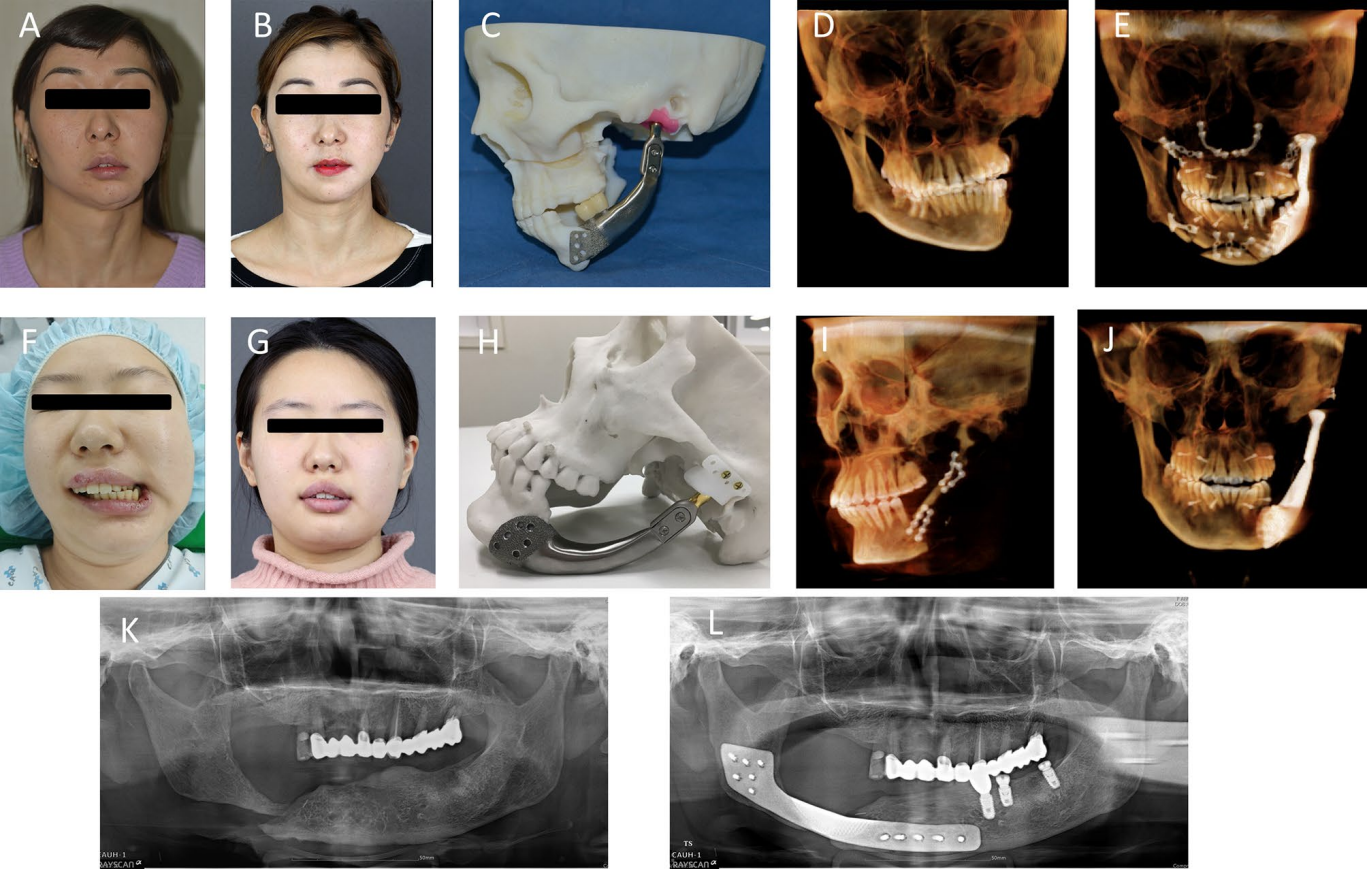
Titanium reconstruction of tumor-induced mandibular defects. (A) A 25-year-old female patient was referred for the reconstruction of the left mandible and correction of facial asymmetry; (B) Postoperative photograph of the female patient. (C) A titanium three-dimensional (3D)-printed mandible was fabricated. The two abutments that faced the central fossa of the maxillary molar were designed for prosthetic restoration. Temporary crowns were also 3D-printed. (D, E) Preoperative and postoperative 3D reconstructed cone-beam computed tomography (CBCT) images. Reproduced from Lee UL et al. J Oral Maxillofac Surg. 2016 Jul;74(7):1501.e1-1501.e15, with permission of Elsevier Inc. (F) A 26-year-old female patient whose mandible was deviated to the left side by more than 10 cm. (G) Postoperative facial photograph shows that the face is symmetric, and the mandible is no longer shifted to left. (H) To overcome these problems, a titanium 3D-printed mandible was fabricated by Medyssey Inc. The Korean Food and Drug Administration does not approve patient-specific joints as of now. Hence, we connected a prefabricated temporomandibular joint to a 3D-printed mandible. (I, J) Preoperative and postoperative 3D reconstructed CBCT images. (K) A 78-year-old-female patient had a right segmental mandibular defect after a mandibulectomy due to malignancy; (L) the mandible was successfully reconstructed with a titanium 3D-printed implant.
